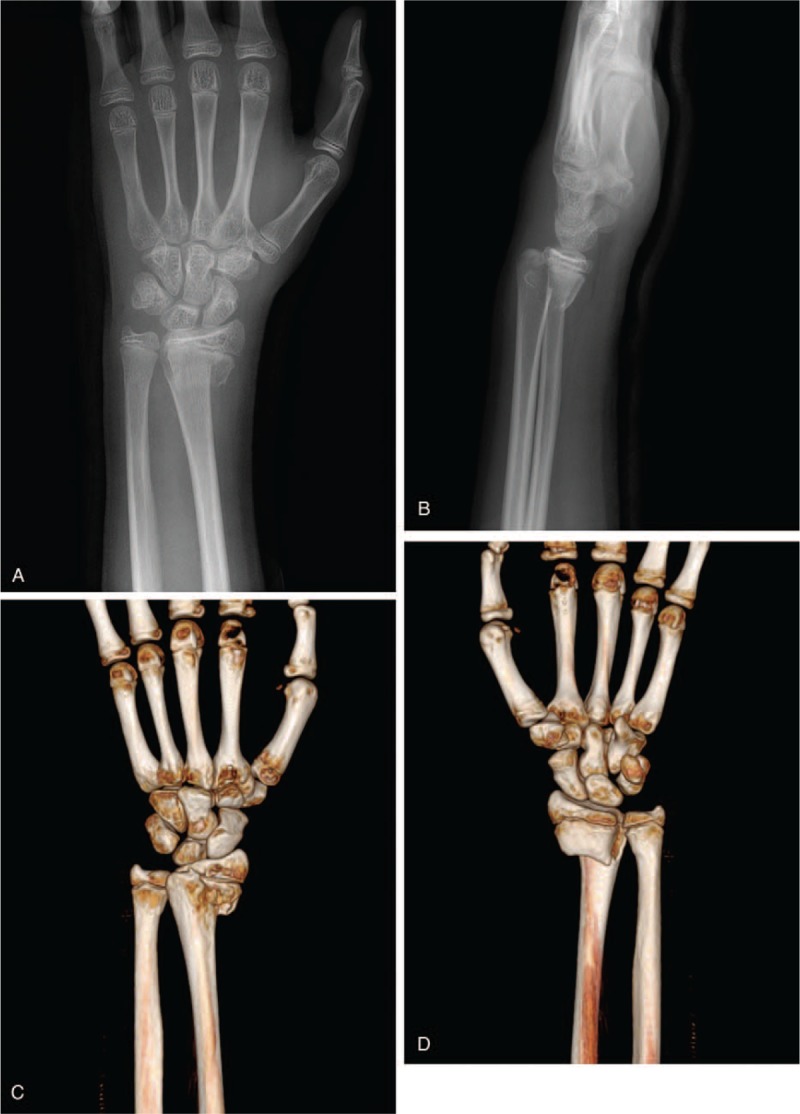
Initial plain radiographs (A, B) and 3D-CT reconstruction images (C, D) showing a displaced Smith fracture with a Salter-Harris type 2 growth plate injury.
